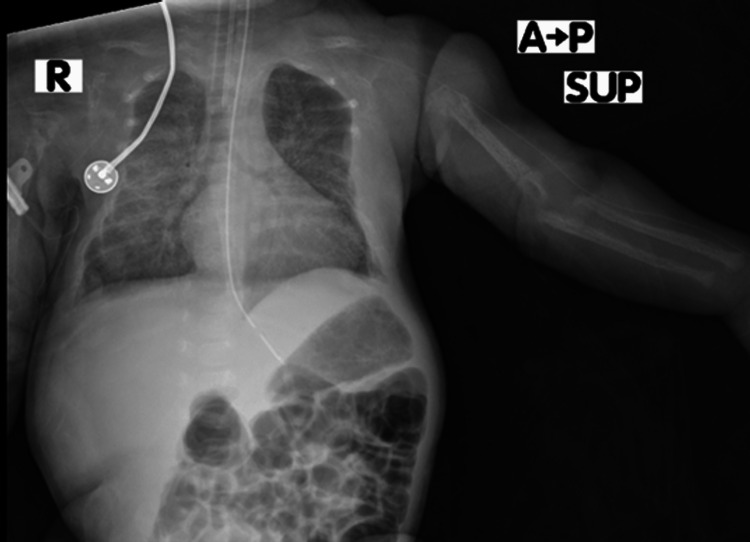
Chest x-ray showing a bell-shaped chest
